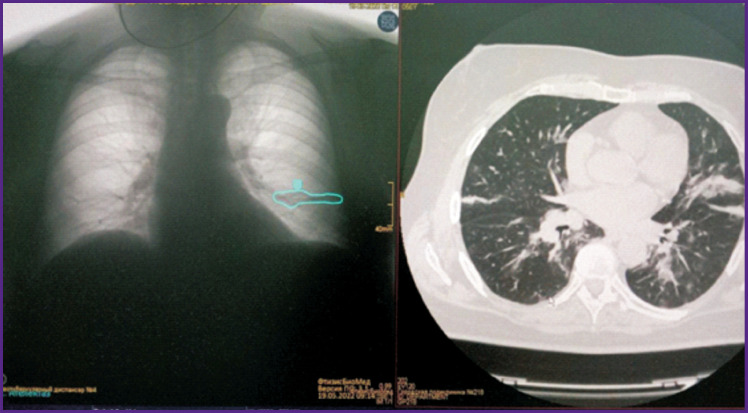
Detection of discoid atelectasis with the help of PhthisisBioMed artificial intelligence (*left*) and CT scan results (*right*)
